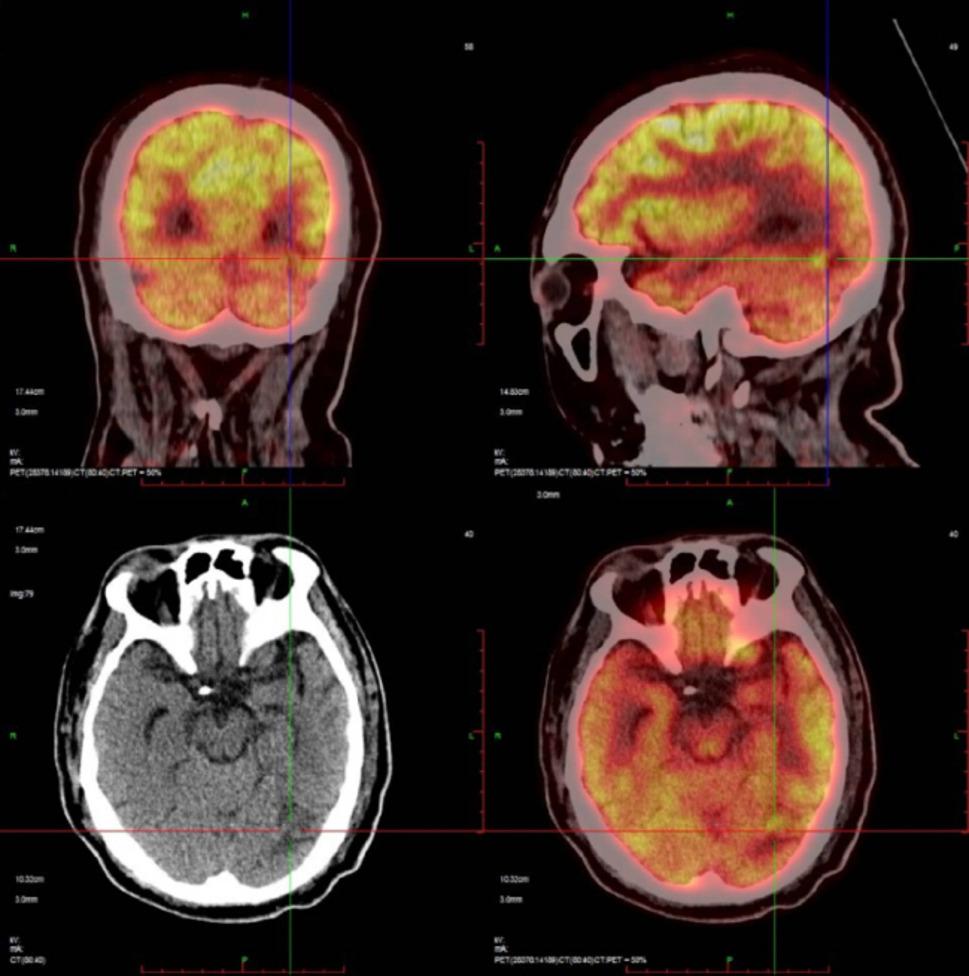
PET-CT imaging demonstrates a hypodense lesion in the left occipital lobe (arrows), representing CNS involvement. This finding correlates with the patient’s visual hallucinations and indicates advanced disease
